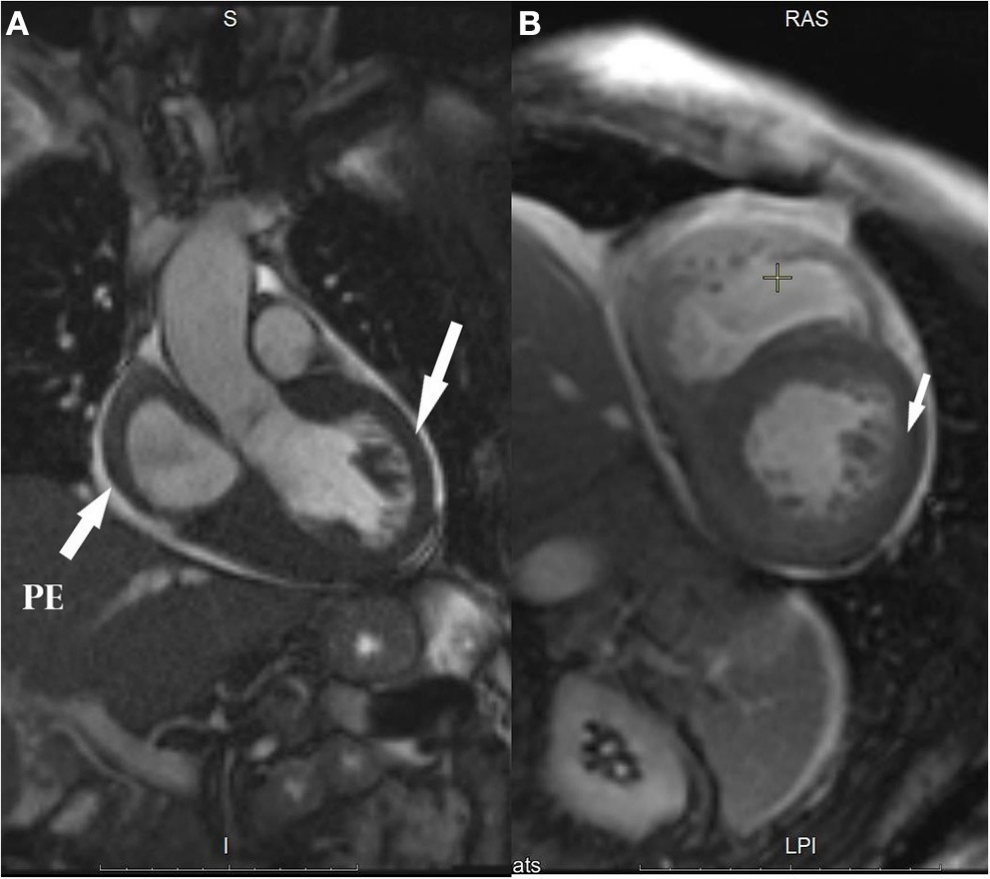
Cardiac MRI images: (A) Left ventricle (LV) outflow tract (arrows show ventricular hypertrophy and pericardial effusion, PE); (B) Two-chamber views (arrow shows global subendocardial hyperenhancement).
